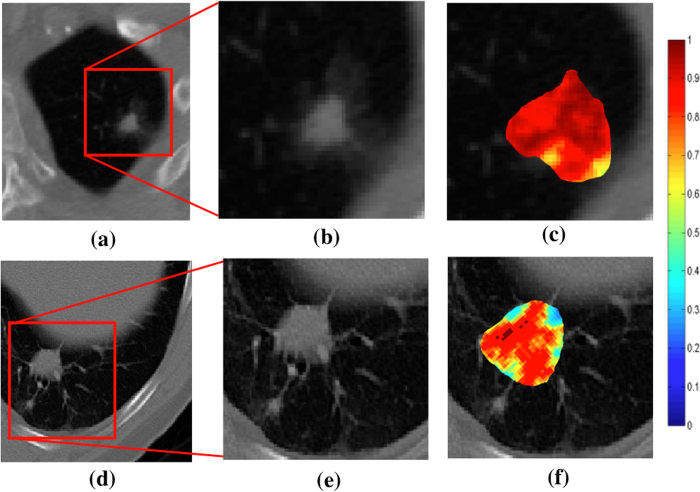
CoLlAGe entropy maps on lung cancer cases. Qualitative feature maps of 2D CoLlAGe visualized on non-contrast lung CT for (a) adenocarcinoma and (d) granuloma shown in (c) and (f) respectively. Adenocarcinomas have higher CoLlAGe entropy values as compared to granulomas. Red represents higher while blue represents low CoLlAGe values.
